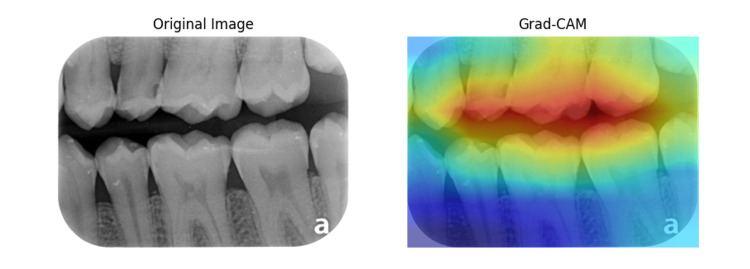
Grad-CAM output images of Bitewing
